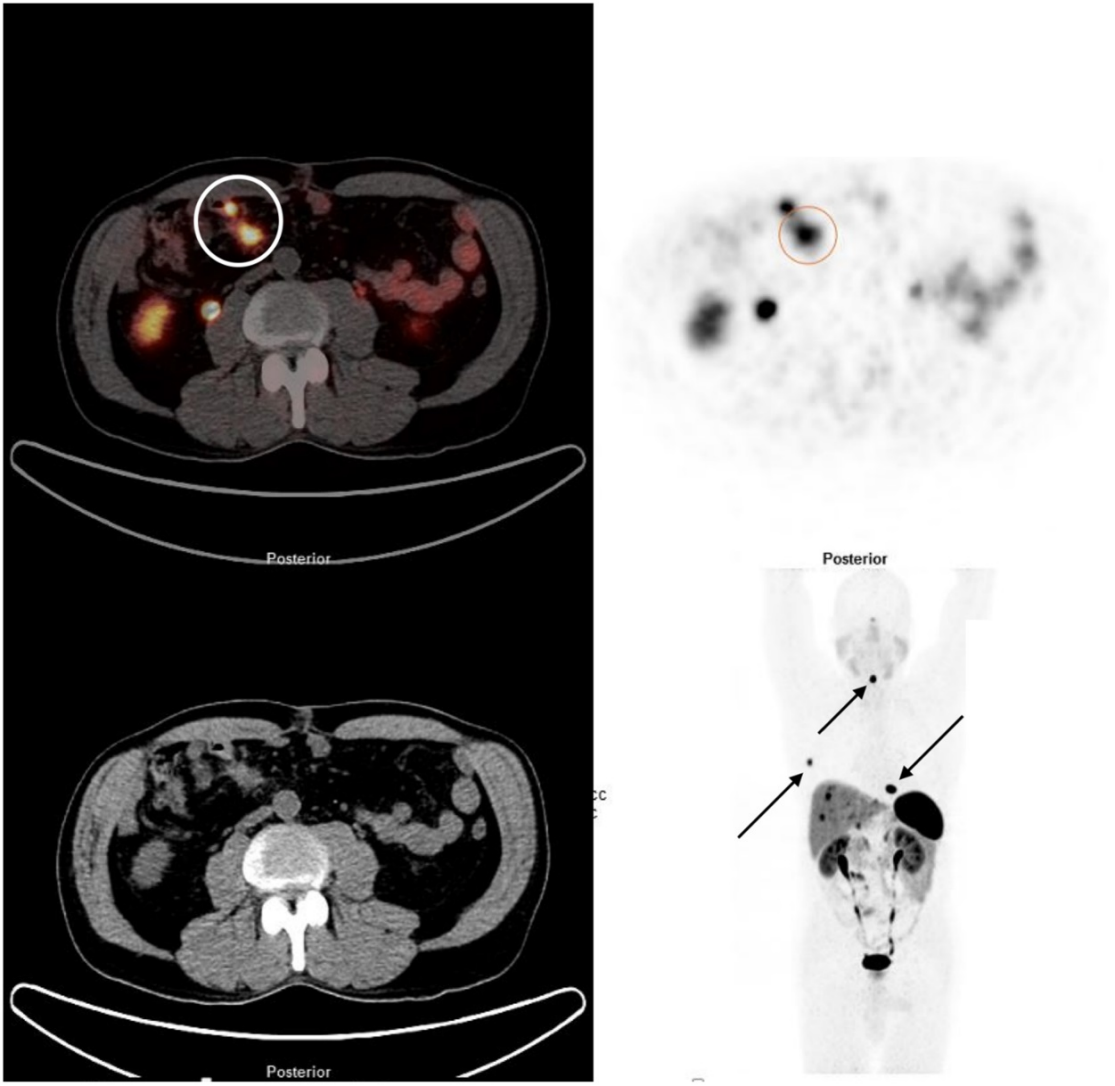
^68^Ga DOTATATE PET/CT demonstrating a SBNEN with lymph node metastases and distant metastases. The primary tumour and locoregional lymph node metastases (stage I) are marked with a circle on the fused image (left side) and on the PET component (right side). The patient also had multiple bone metastases (rib, cervical spine and thoracic spine [arrows]) and liver metastases.
